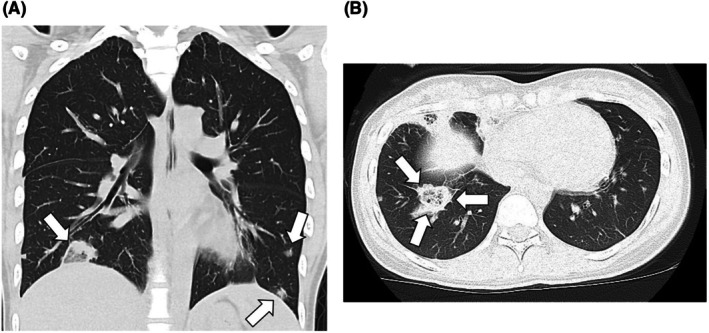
Computed tomography at admission. (A) Multiple infiltrative and nodular shadows were observed along the pleura of both lungs. (B) A reversed halo sign was noted in the right middle and lower lung fields.
